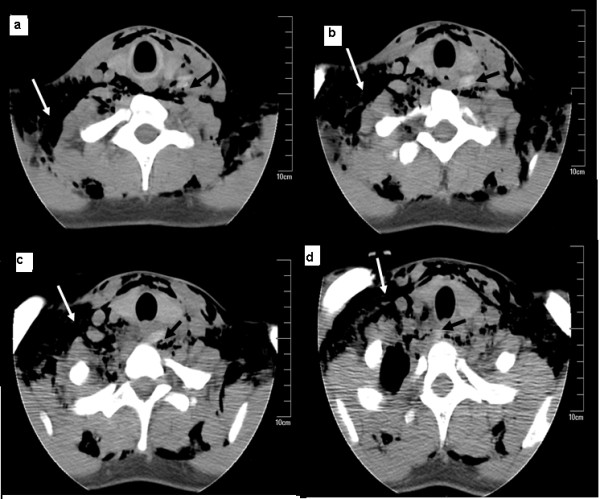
Plain computed tomography scan of the neck (a,b,c,d) obtained on the third day after the accident. The computed tomography scan shows extensive subcutaneous emphysema in the neck and upper breast (white arrowhead). A dense shadow is visualized as a linear structure (black arrowhead) at the level of the 7th cervical vertebra and 1st dorsal vertebra pointing towards the common carotid artery.
